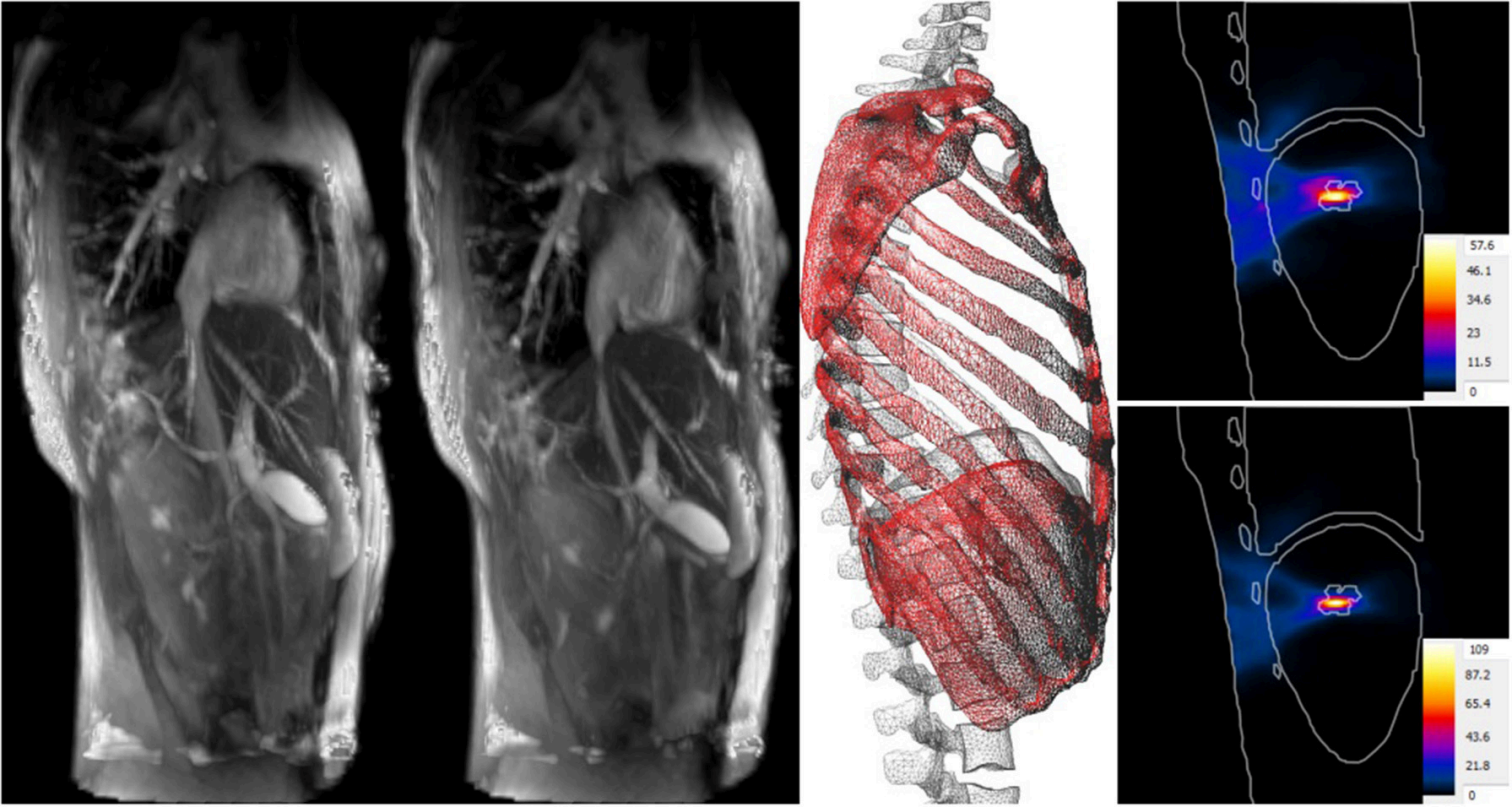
Impact of breathing motion on focused ultrasound liver ablation. 4D MRI data (A at maximal exhalation, B at maximal inhalation) is used to create deformation fields that are registered to the anatomical model, thus functionalizing it with breathing motion (C). Compared to the temperature increase when neglecting motion (D), tracking compensation (E) can double the theoretical temperature increase, result in better focusing, and reduce collateral damage.
